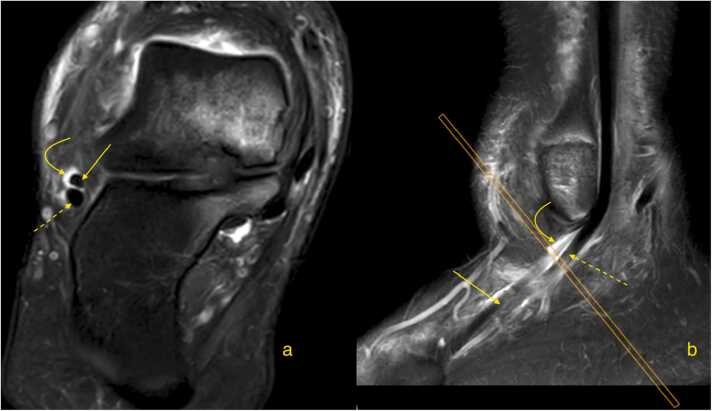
A patient with a recent ankle injury presenting with joint pain. Magnetic resonance imaging shows: (a) Proton density-weighted image with fat suppression in an oblique section (the level of the section is indicated on image b), and (b) Proton density-weighted image with fat suppression in a coronal section. Effusion in the common peroneal synovial sheath (curved arrow) is more prominent inferior to the lateral malleolus. The peroneus brevis (straight arrow) and peroneus longus (straight dashed arrow) tendons appear normal.
